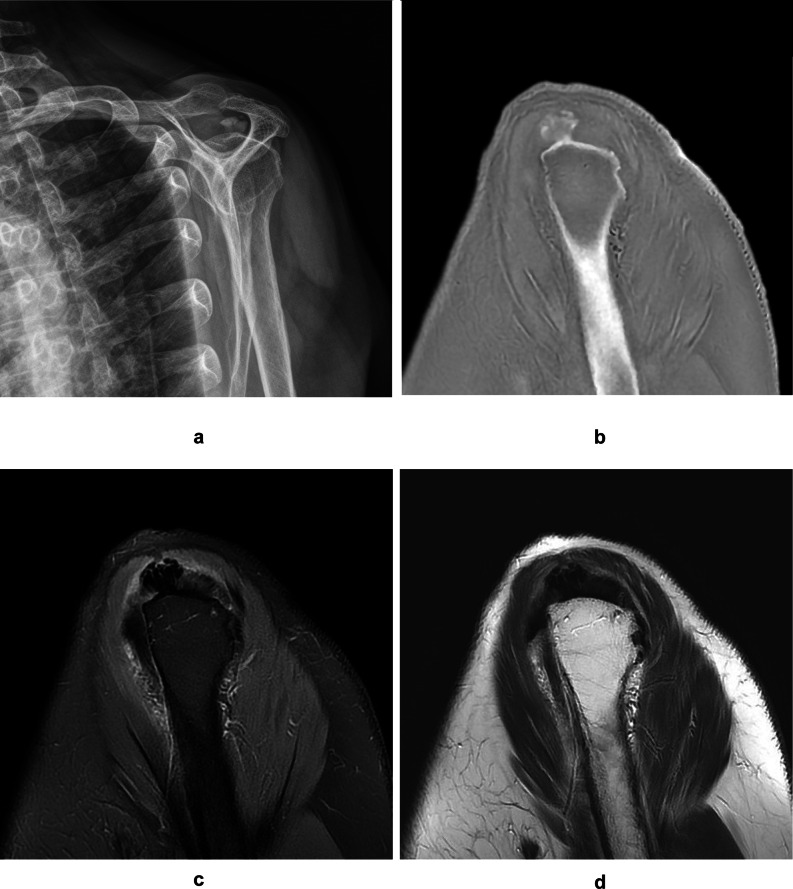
A 85-year-old patient with calcific tendinitis in resorptive phase at supraspinatus tendon of shoulder. Radiography (A) showed a poorly defined, fluffy, and amorphous type 1 calcific deposit by Depalma’s classification. Sagittal ZTE image (B) showed a irregular shaped, ill-defined calcific deposit with heterogeneous low signal intensity. Sagittal FSPDWI (C) showed a irregular shaped calcific deposit with low signal intensity. Note high signal intensity surrounding calcific deposit and subacromial-subdeltoid bursa representing inflammation (arrow). A calcific deposit on sagittal T2WI (D) showed similar low signal intensity with surrounding supraspinatus tendon which results in ill-defined margin and smaller size.
